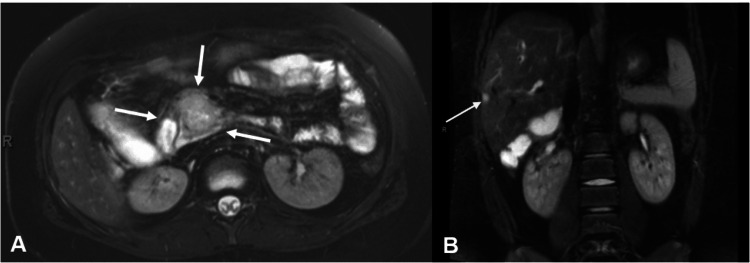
MRI of the abdomen with contrast demonstrates a pancreatic head mass (A) and solitary metastatic lesion to the liver (B) MRI: magnetic resonance image
